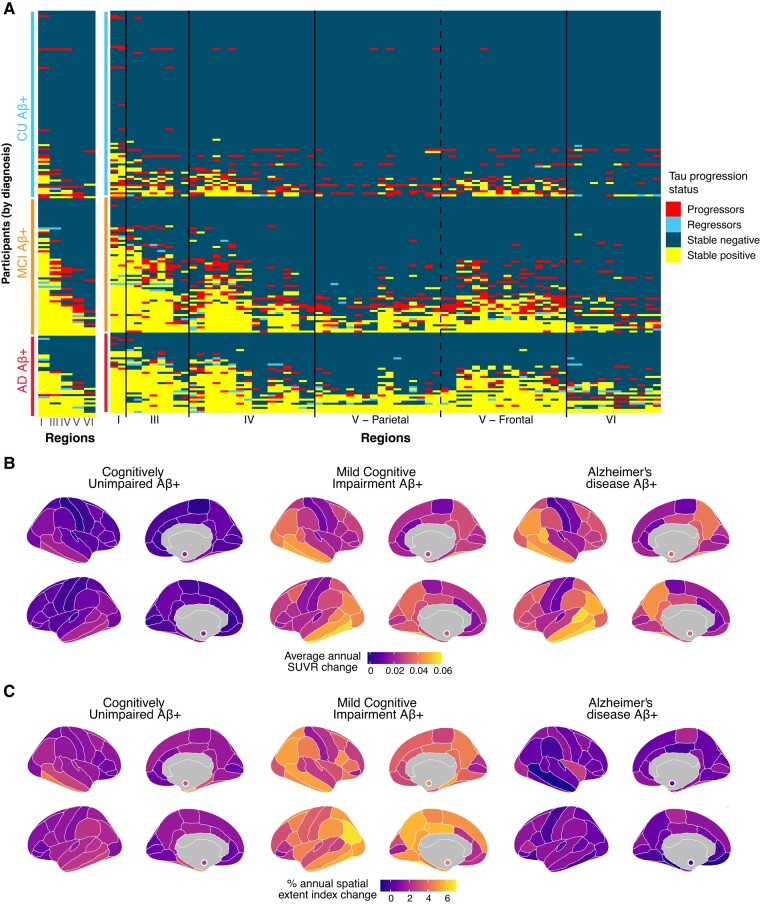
Spatial localization of abnormal tau accumulation over time in amyloid-positive participants of the ADNI cohort. (A) Abnormal accumulation is presented by (I) Braak stages and (II) all 70 individual brain regions of the Desikan atlas. Colours denote the change in the region between the baseline and the last available visit. A stable region (negative or positive) did not change status during the follow-up. A progressing region was originally negative and subsequently became positive over time. A regressing region was originally positive and became negative over time. (B) Brain maps presenting the average SUVR change per region per year. (C) Brain maps representing the percentage of participants becoming tau-positive in each region annually. In both B and C, values in the bilateral amygdalae are represented by small coloured circles in the medial view of the brain, and the annual change is calculated in each region using linear mixed-effect models with random slopes and intercepts. Only participants with at least three tau scans (*n* = 100) were kept for B and C to ensure a constant sample across the longitudinal follow-ups.
